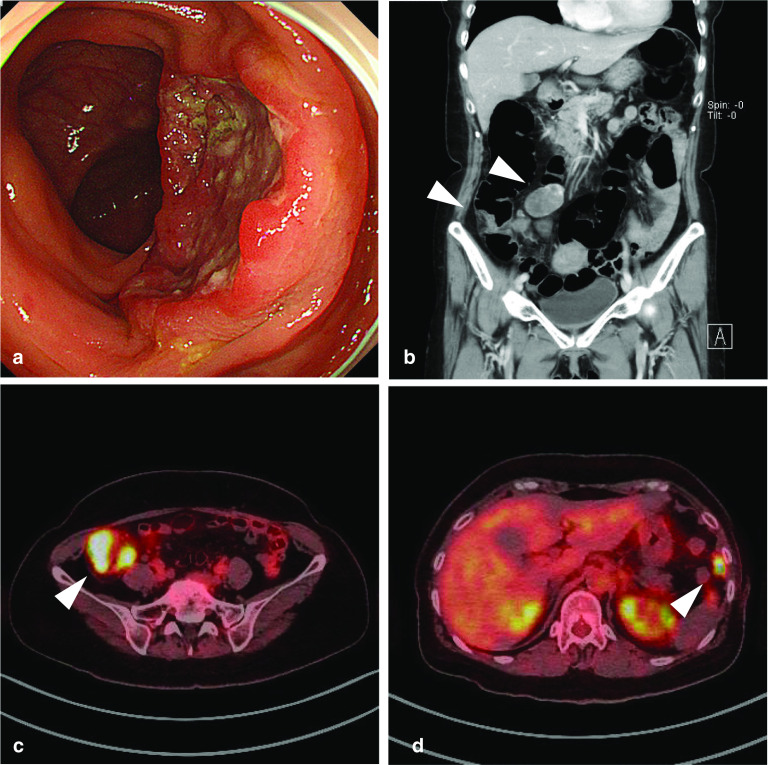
a Colonoscopy showed a semicircular type 3 tumor in the ascending colon. b CT showed a mass in the ascending colon with regional lymph node enlargement (arrowheads). c, d FDG-PET showed abnormal FDG uptake in the lesion in the ascending colon and peritoneal thickening of the left upper abdomen
